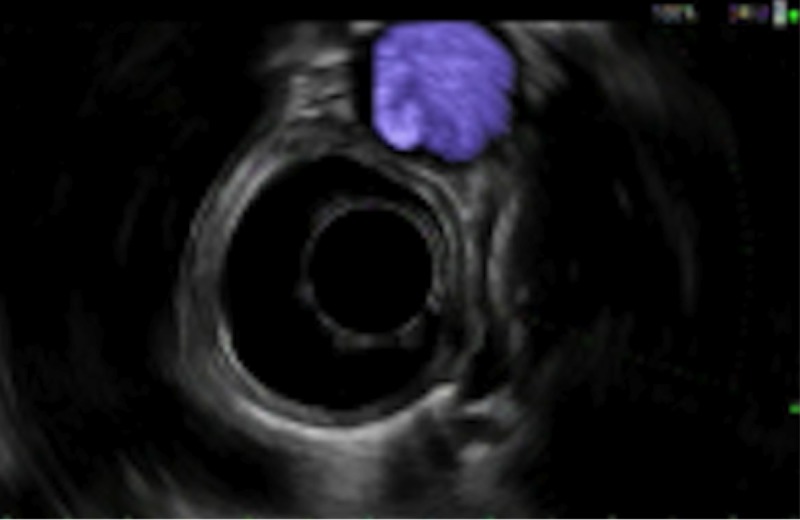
Sonographic image of the nodule on EUS Intramural, sub-epithelial nodule located within the deep mucosa of layer 2 on endoscopic ultrasound (EUS).
